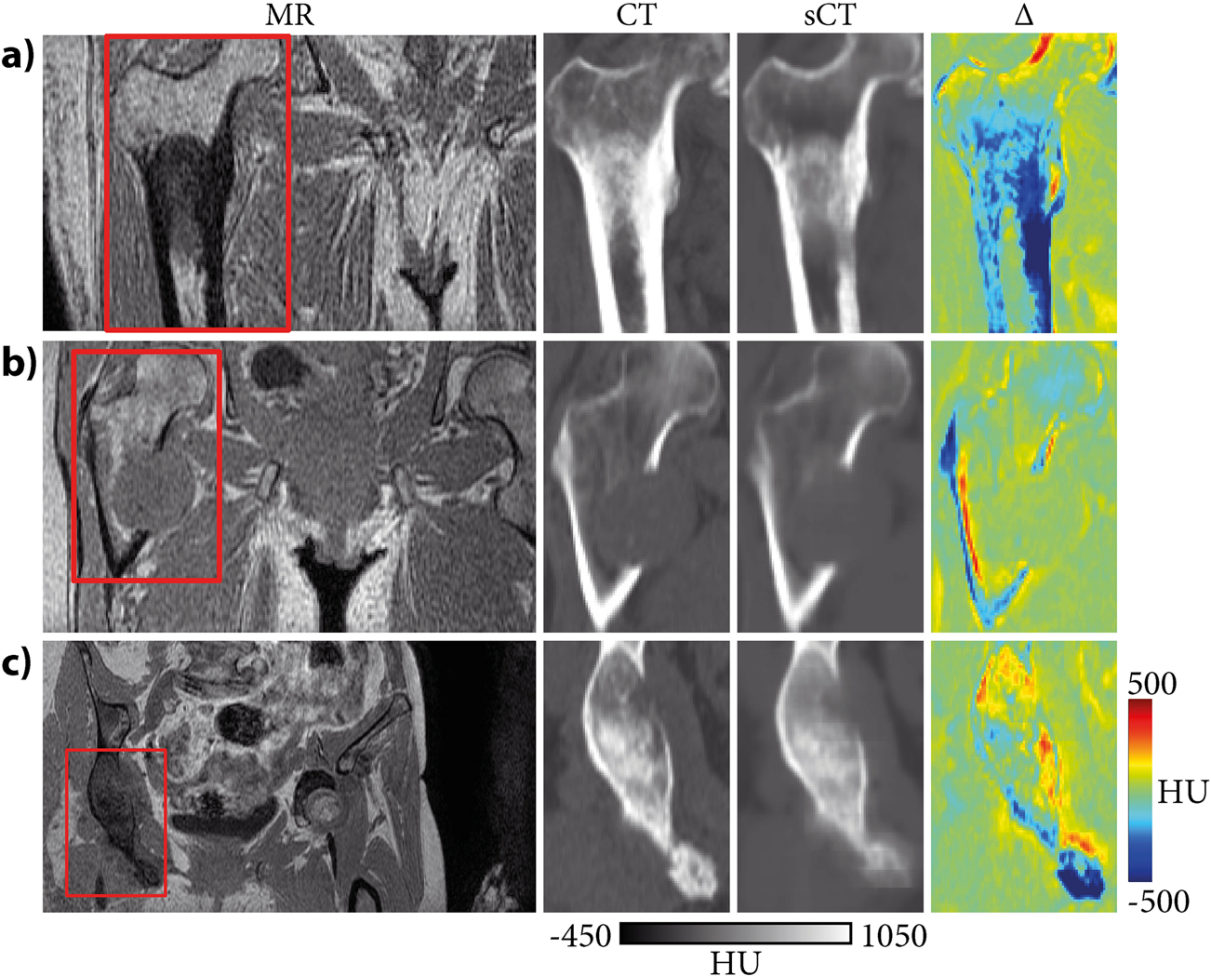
MR, CT, synthetic CT (sCT), and sCT-to-CT difference (Δ) obtained for three patients presenting (a) osteoblastic, (b) osteolytic, and (c) mixed lesions. Red boxes on the MR images indicate the region that was zoomed in to compare CT and sCT images
